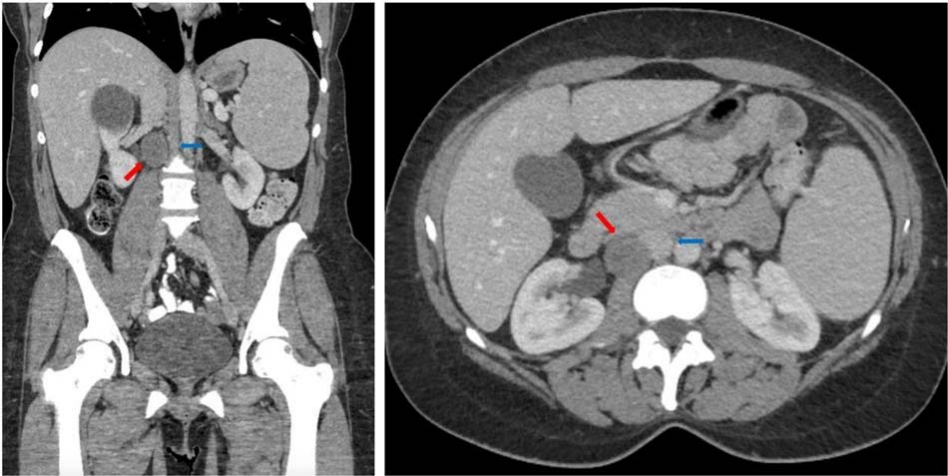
Coronal (left) and axial (right) CT of the abdomen and pelvis showing the retrocaval schwannoma (red arrow) and IVC (blue arrow).
